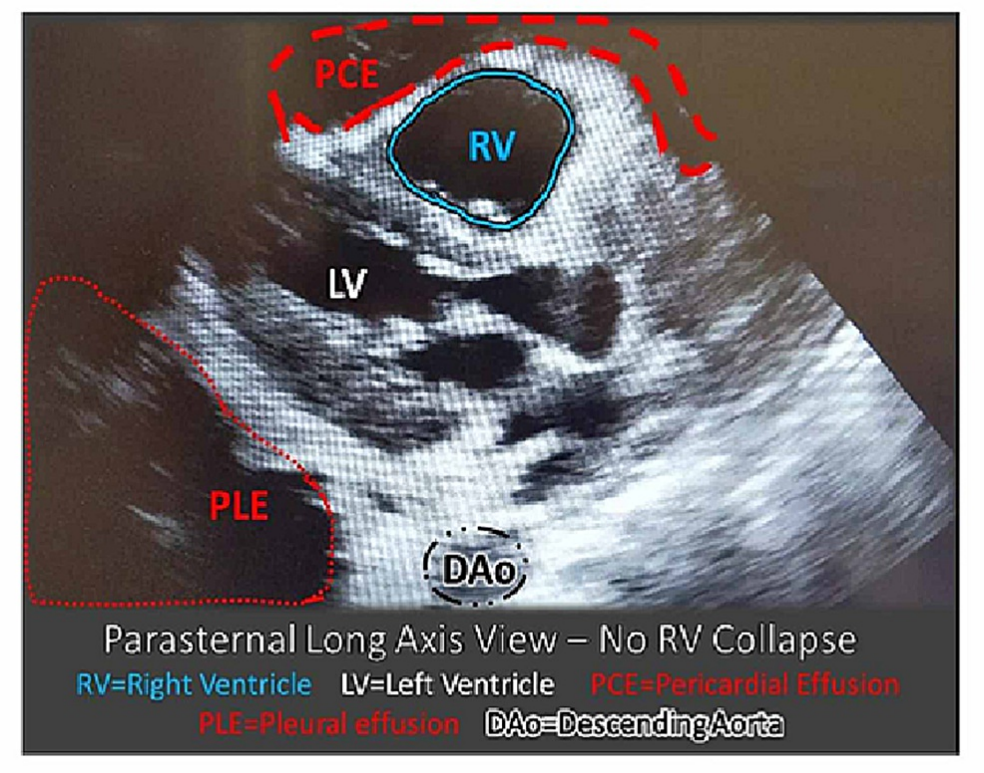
POCUS of the heart parasternal long-axis view – note the RV is not collapsed. There is a small PCE. A PLE is noted as it is posterior to the DAo. POCUS: point-of-care ultrasound; PCE: pericardial effusion; PLE: large pleural effusion; DAo: descending aorta; RV: right ventricle
